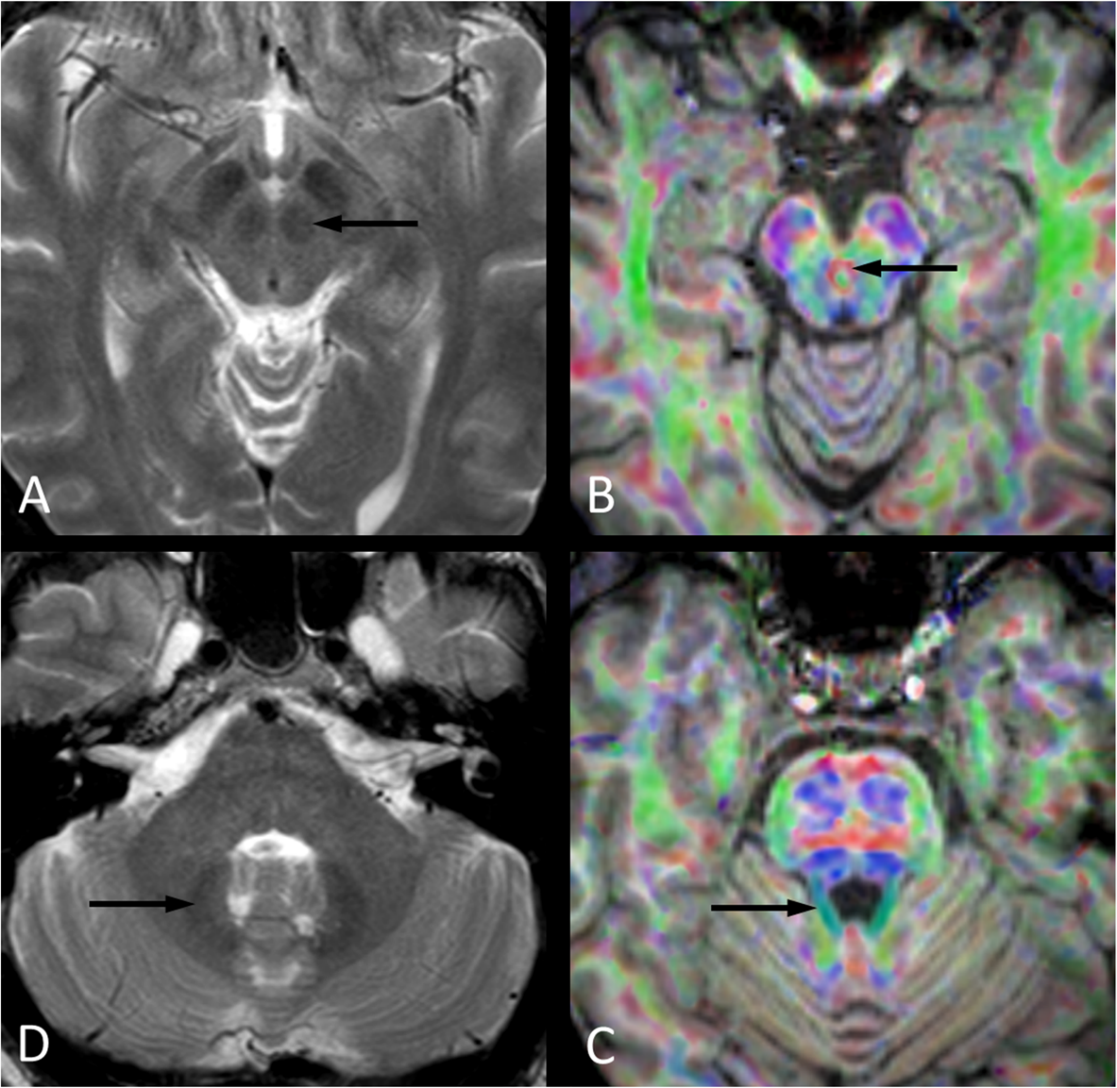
Most important anatomical structures involved in the cerebrocerebellar neurocognitive regulatory system; axial T2-weighted magnetic resonance images at the level of the midbrain (A) and brachium pontis (D) and cross-sectional color maps derived from diffusion tensor imaging (DTI) with T1-weighted anatomical information at the level of the interpeduncular fossa (B) and the pons (C). The cerebellorubral tract connects the dentate nucleus (D, arrow) with the contralateral red nucleus (A, arrow). Its fibers are a component of the brachium conjunctivum (C, arrow) and cross the midline via the decussation (B, arrow) of the superior cerebellar peduncles (brachia conjunctiva) just below the level of the inferior colliculi.
